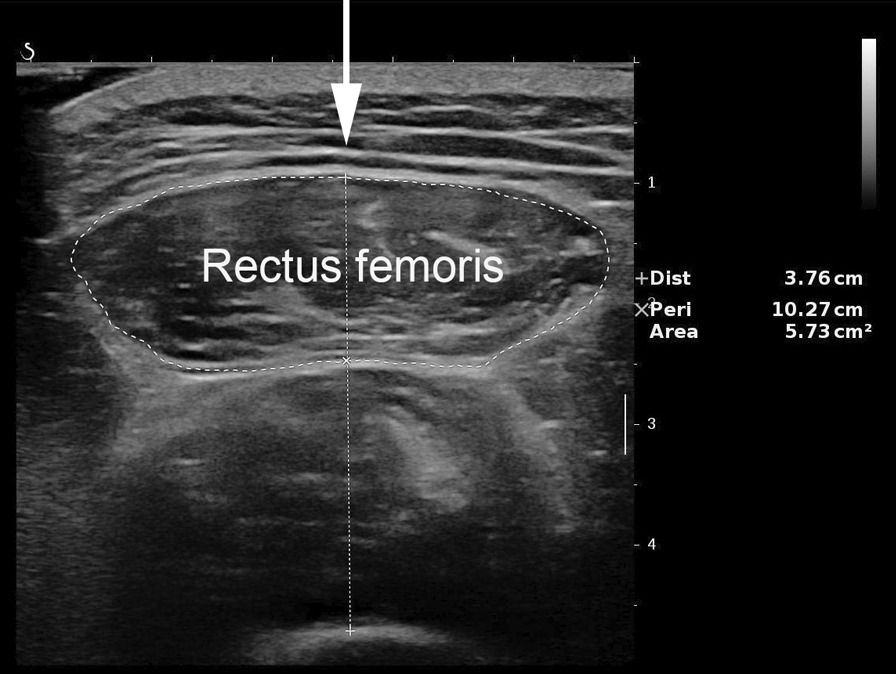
Ultrasound of the rectus femoris. The arrow indicates the direction of the scan
